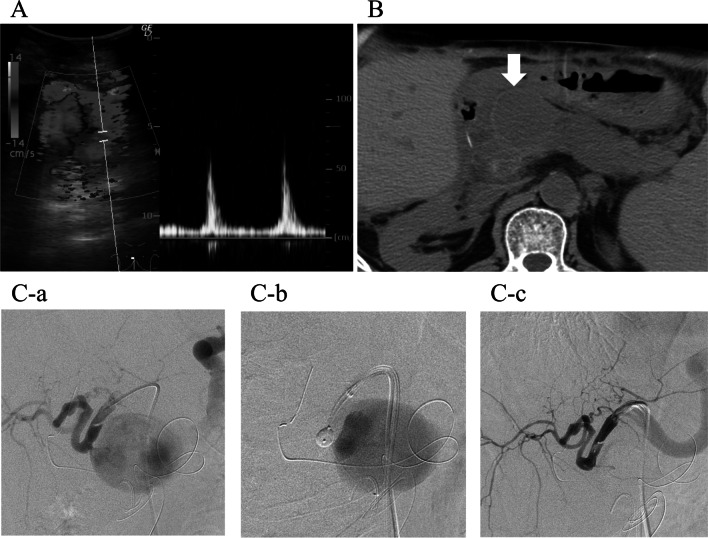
Diagnosis of the HAP in the CHA 8 months after LDLT. A Abdominal ultrasonography revealing a 4-cm mass with a to-and-fro wave pattern at the neck of the lesion on Doppler. B Plain abdominal computed tomography imaging showing a 4-cm cystic lesion in the area of the pancreatic head. C-a Celiac angiography revealing a narrow-neck type HAP in the CHA and a tortuous PHA. C-b Under balloon protection of the PHA, thrombin was injected into the HAP. C-c Post-procedure angiography showing complete occlusion of the HAP and a good flow to the graft liver. *CHA* common hepatic artery, *HAP* hepatic artery pseudoaneurysm, *LDLT* living donor liver transplantation, *PHA* proper hepatic artery
